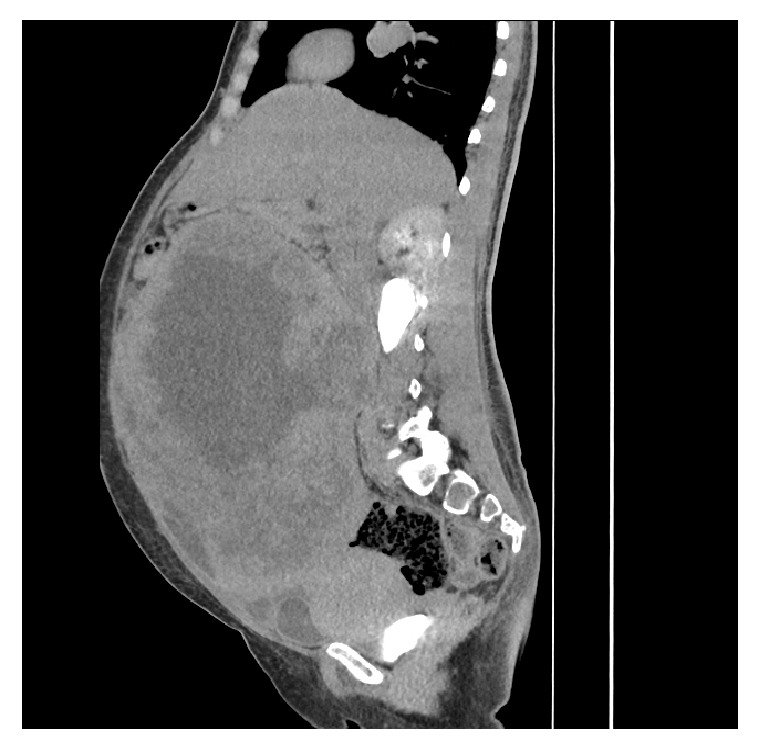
Presurgical evaluation showing the sagital slice of the abdominopelvic CT scan.
